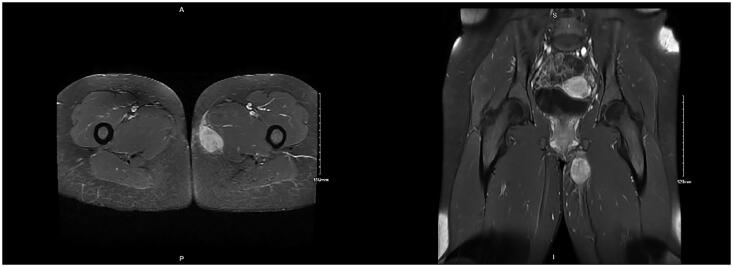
MRI demonstrating soft tissue mass measuring 4.8 x 2.7 x 4.0 cm extending into the left gracilis muscle.
